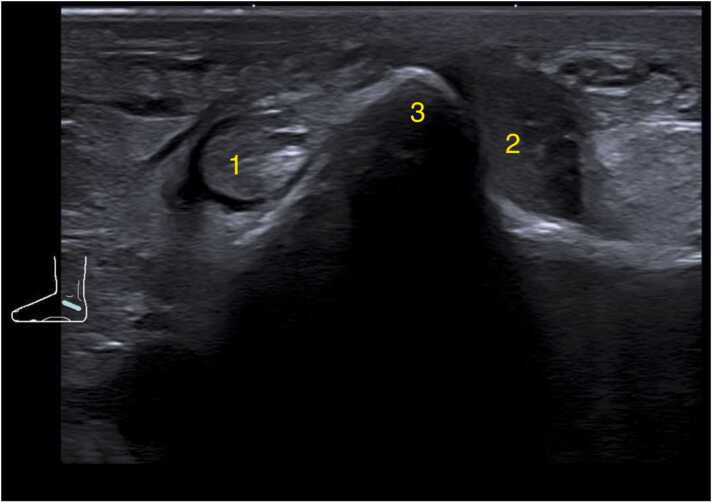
Ultrasound oblique cross-section at the level of the peroneal tubercle. The peroneus brevis tendon runs over the tubercle, while the peroneus longus tendon passes underneath it. 1- Peroneus longus, 2- Peroneus brevis with a small amount of fluid in the synovial tendon sheath, 3- Peroneal tubercle.
